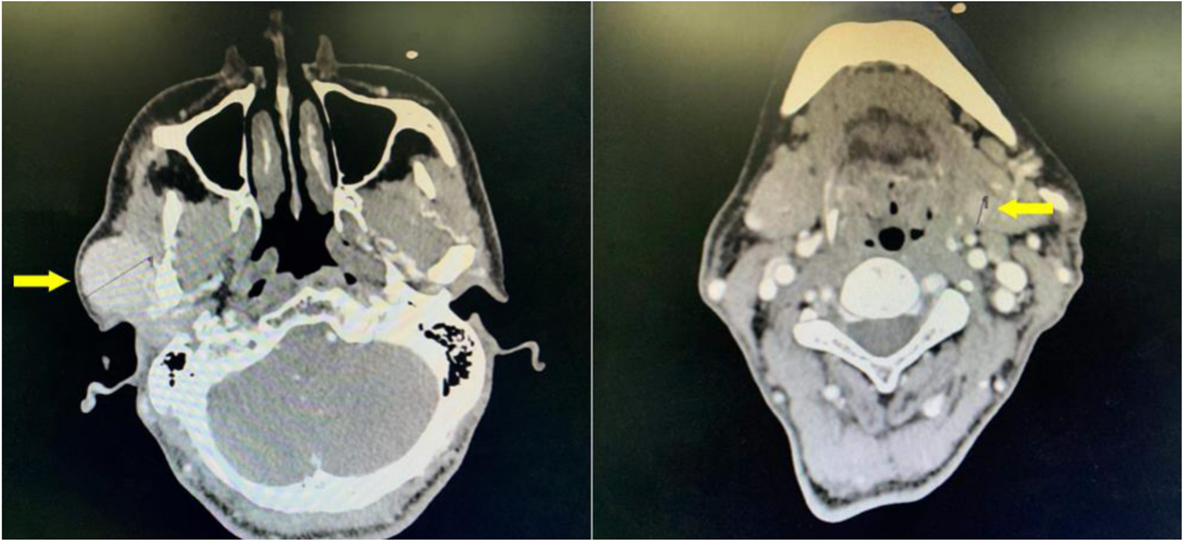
CT scan showed multiple masses in the bilateral parotid and submandibular gland
